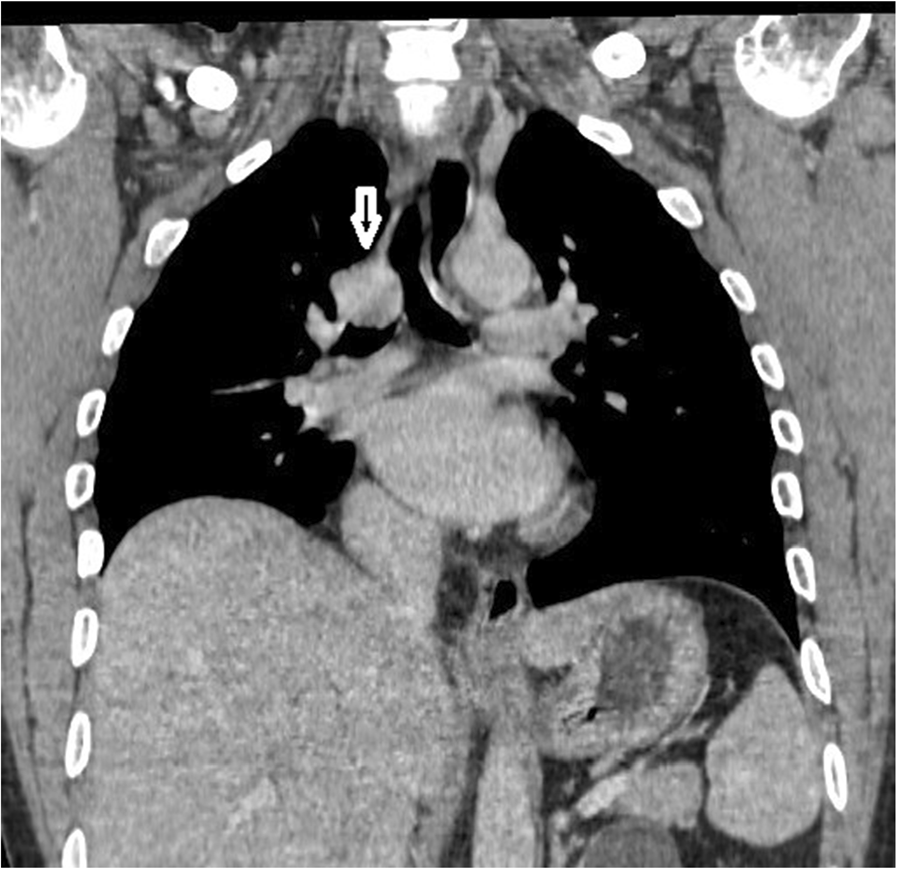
Computed tomography showed a circular mass in the lower trachea (arrow), with extra tracheal development.
